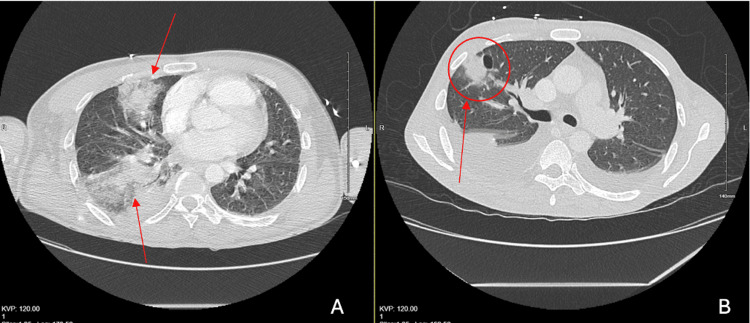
(A) A CT scan of the chest with contrast revealed acute right middle and lower lobar pulmonary emboli with nodular opacities (two red arrows) in the peripheral right upper lobe in the setting of recurrent TV endocarditis. (B) Compared with the CT from two months later, which revealed right middle lobe peripheral opacities (red arrow with circle) suspicious for septic emboli in the setting of TV endocarditis CT: computed tomography, TV: tricuspid valve
